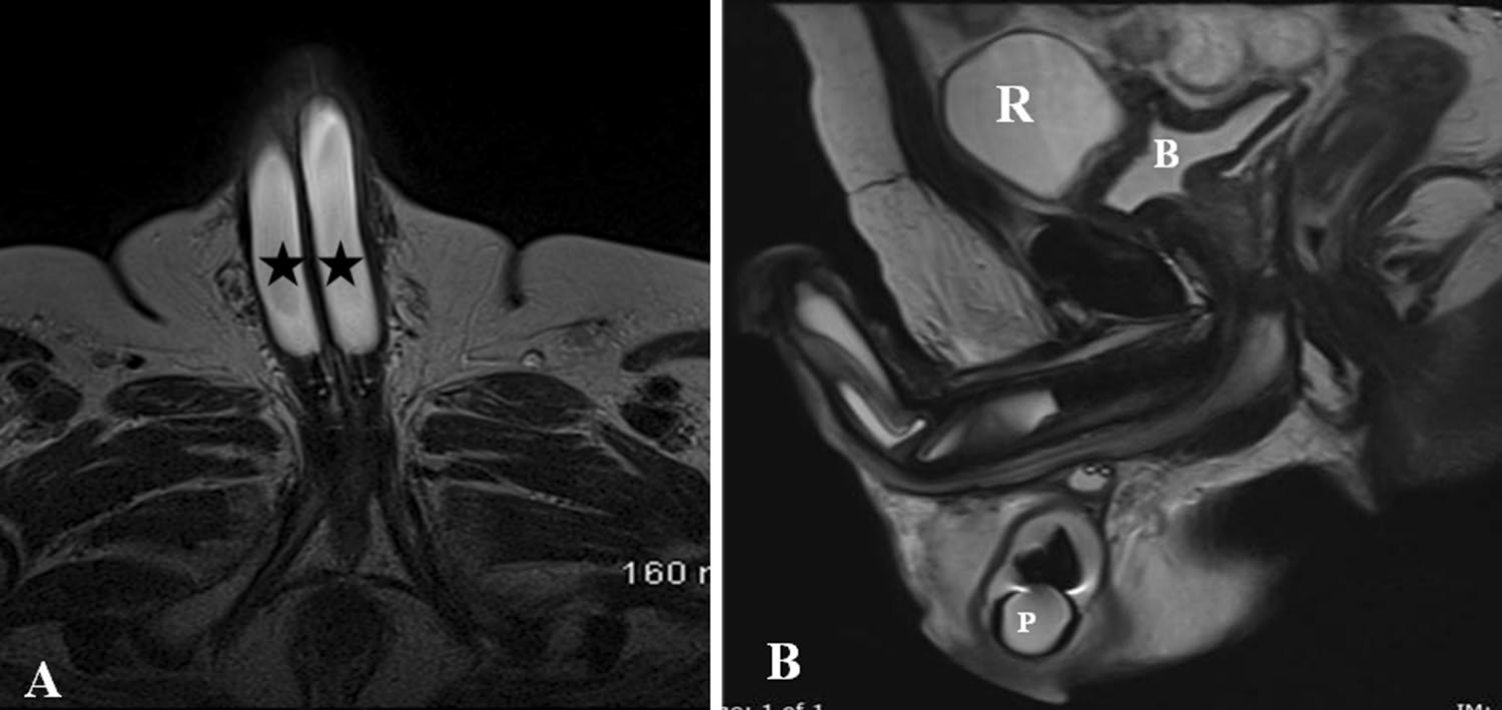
Appearance of normal inflatable penile prosthesis in a 65-year-old diabetic male. a Axial T2-weighted MRI shows the fluid filled corporal cylinders (asterisk). b Sagittal T2-weighted MRI shows the pelvic reservoir (R) adjacent to urinary bladder (B) and scrotal pump (P)
